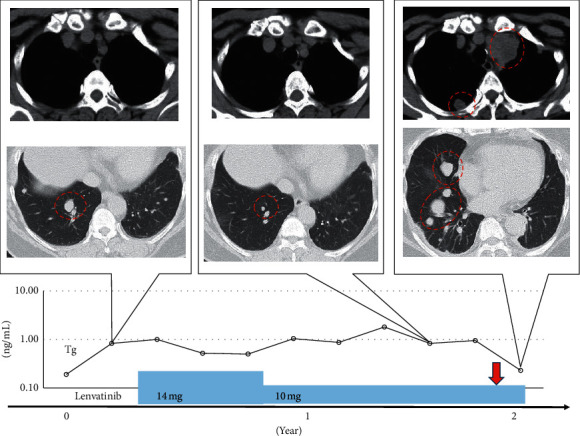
Clinical course of Case 3. The upper image is a chest computed tomography image of the mediastinal condition, and new lesions appeared in the mediastinal lymph nodes and pleura. In the lower image, the target lesion became partial response after lenvatinib treatment; then, it became progressive disease after anaplastic transformation. The graph below the image shows the transition of thyroglobulin, the timing of lenvatinib treatment, and the dose. The red dotted circle indicates the lesion. The red arrow indicates the time of anaplastic transformation.
